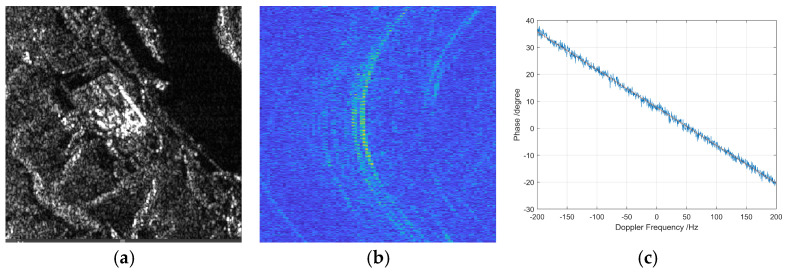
Multi-channel echo range migration curve of single house in the mountains. (a) Image slice amplitude. (b) Range migration curve after range compression in time-domain. (c) Channel phase error varying with Doppler frequency.
